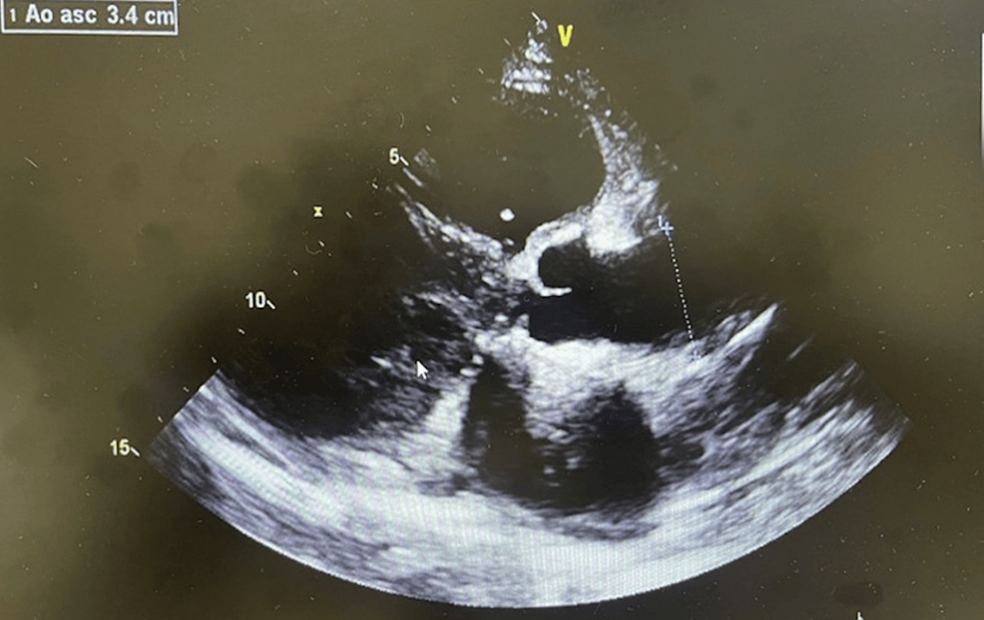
Echocardiogram showing ventricular dilation with ventricular wall hypertrophy.
